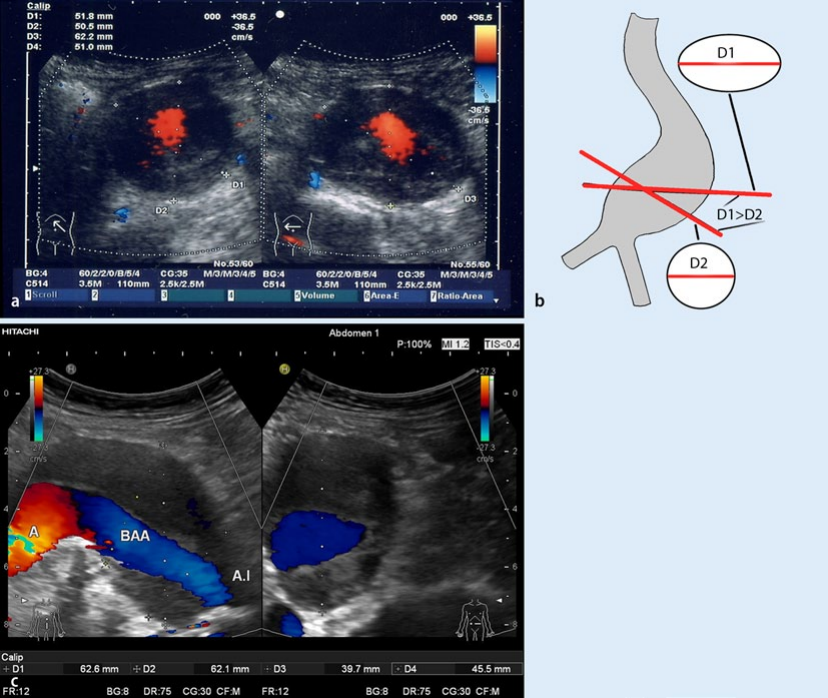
a Aortic diameter in AAA with left-lateral elongation: comparison of the measurement obtained in upper abdominal cross section (*right*; see body marker) of 62.2 mm (*D3*) with the measurement of 51.8 mm (*D1*) when the diameter is turned on the vessel axis at the same point (*left*). The orthogonal diameter measurement (*left*) corresponds to the real diameter. The anteroposterior (AP) measurement remains constant (50.5 and 51 mm in *D2* and *D4, respectively*). b Appropriate diameter measurement in AAA with an elongated vessel course. Measuring in abdominal cross section results in a false-high diameter due to the elliptical representation obtained in oblique section of the aneurysmal sack (*D1*). This measurement often also has low reproducibility, resulting in fluctuations in measured values. In order to obtain appropriate as well as reproducible measurements, the transducer should be turned in the area of maximum diameter in such a way that the real aneurysm transverse diameter (*D2*) perpendicular to the vessel axis is visualized (often a round structure) (modified from [20]). c A comparison of maximum AAA diameter measurement (ventrolateral elongation) of 62.6 mm (*D1*) in abdominal cross section in the AP plane (*right*) with measurement in the orthogonal plane (perpendicular to the vessel axis in longitudinal section; *left*). The real aortic diameter here is only a maximum of 45.5 mm (*D4*); at the measurement point in the AP transverse plane, it is only 39.7 mm (*D3*). The measurement of 62.1 mm in cross section (*right*) is also marked with measuring marks (*D2*) in the longitudinal section (*left*). Diameter measurement in the AP plane in the *right-hand* section of the image corresponds to the AP measurement on CT in an axial plane (without reconstruction). *BAA* abdominal aortic aneurysm, *A.I* iliac artery
